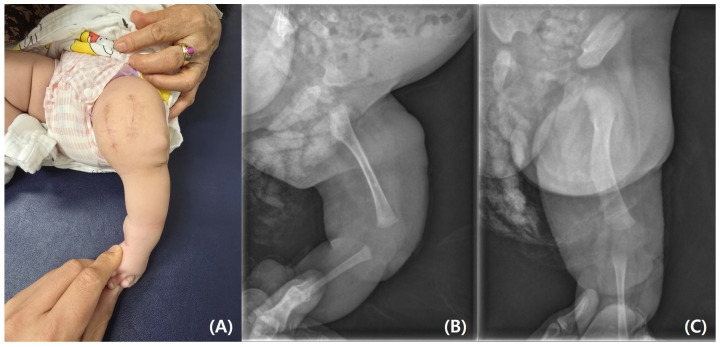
(A) Postoperative photograph. (B) Postoperative lateral and (C) anteroposterior radiographs.
